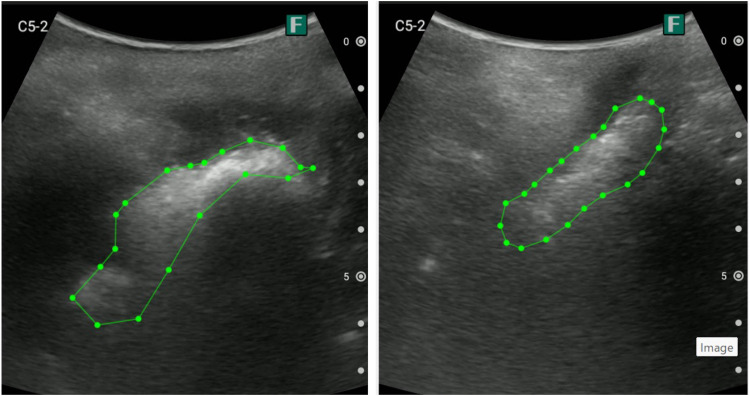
Examples of annotations made by sonographers on ultrasound images. Hyperechoic areas indicating fecal retention in the lower rectum are surrounded by polygons using the annotation tool LabelMe on a PC.
